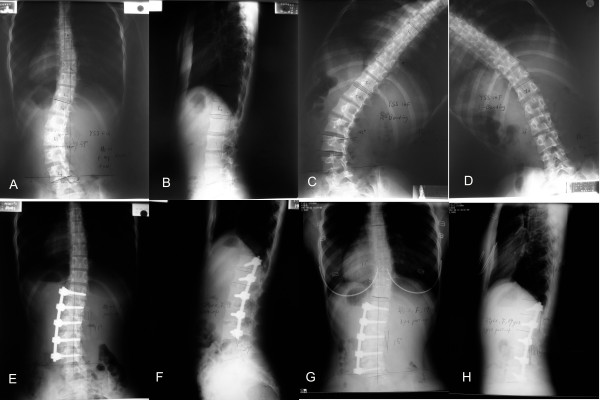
A 16-year-old female, AIS, PUMC IId1 type. Preoperative X-ray showed a 39° left lumbar curve and a 20° right thoracic curve and the apex of the lumbar curve was located at L_2,3 _disc. Preoperative lateral X-ray showed no thoracolumbar kyphosis (A, B). The interspace angle between L_4 _and L_5 _was 4°. On the right Bending film, the interspace angle was 9° (C). On the left Bending film, it turned to 0° (D). Anterior correction and fusion was performed, and the fusion level was from T_12 _to L4. Postoperative films showed a good correction and the interspace angle was improved to 0° (E, F). Three-year post-operative follow-up, the interspace angle increased to 2° with also a good coronal balance(G, H).
